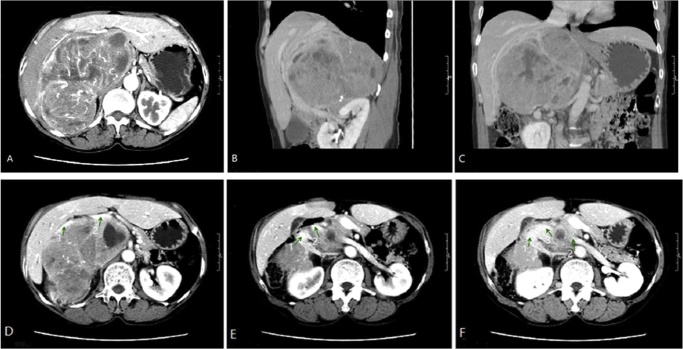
The CT images of the tumor where venous involvement was considered. Fig. 1D: inferior vena cava(arrow 1), portal vein (arrow 2); Fig. 1E: right renal vein(arrow 1), left renal vein(arrow2); Fig. 1F:right renal vein(arrow 1), left renal vein(arrow2), inferior vena cava(arrow 3).
